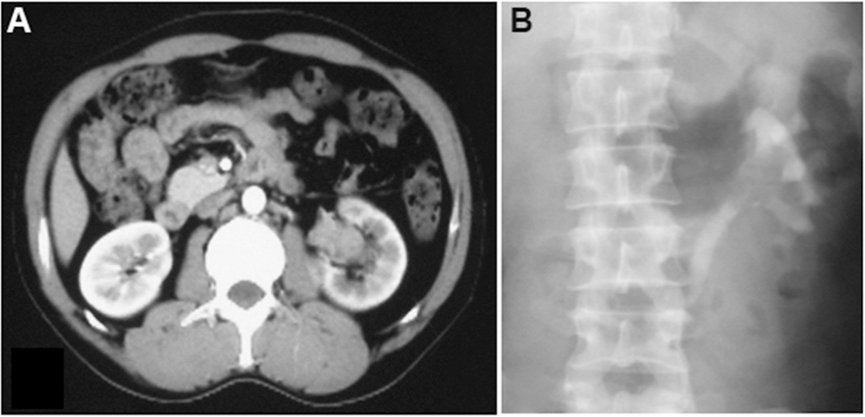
Contrast-enhanced CT demonstrating a 4-cm soft-tissue mass occupying the dilated left renal pelvis. (A) Enhanced CT. (B) Left retrograde pyelography.
